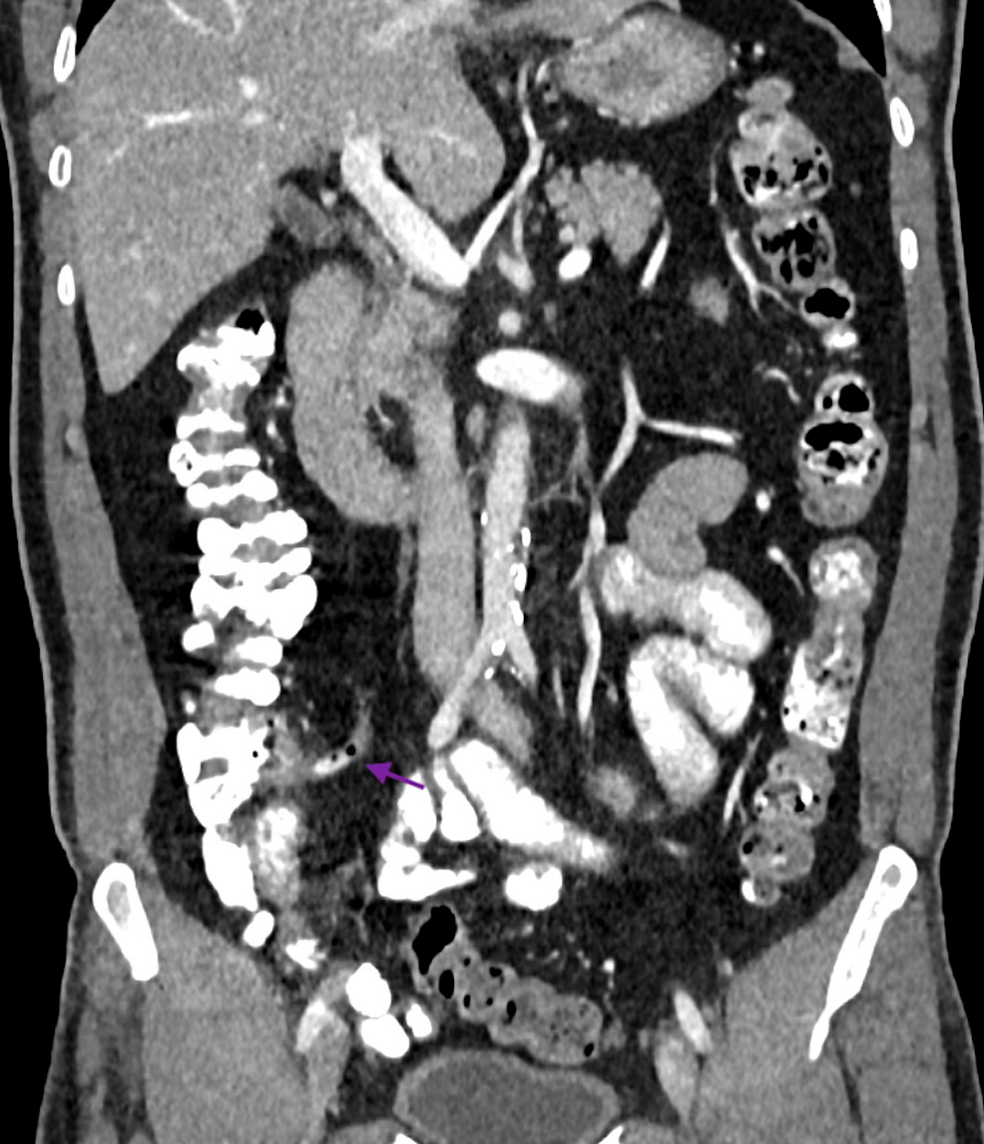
Coronal section of contrast-enhanced CT abdomen showing normal appendix (purple arrow) with intraluminal air and contrast.
